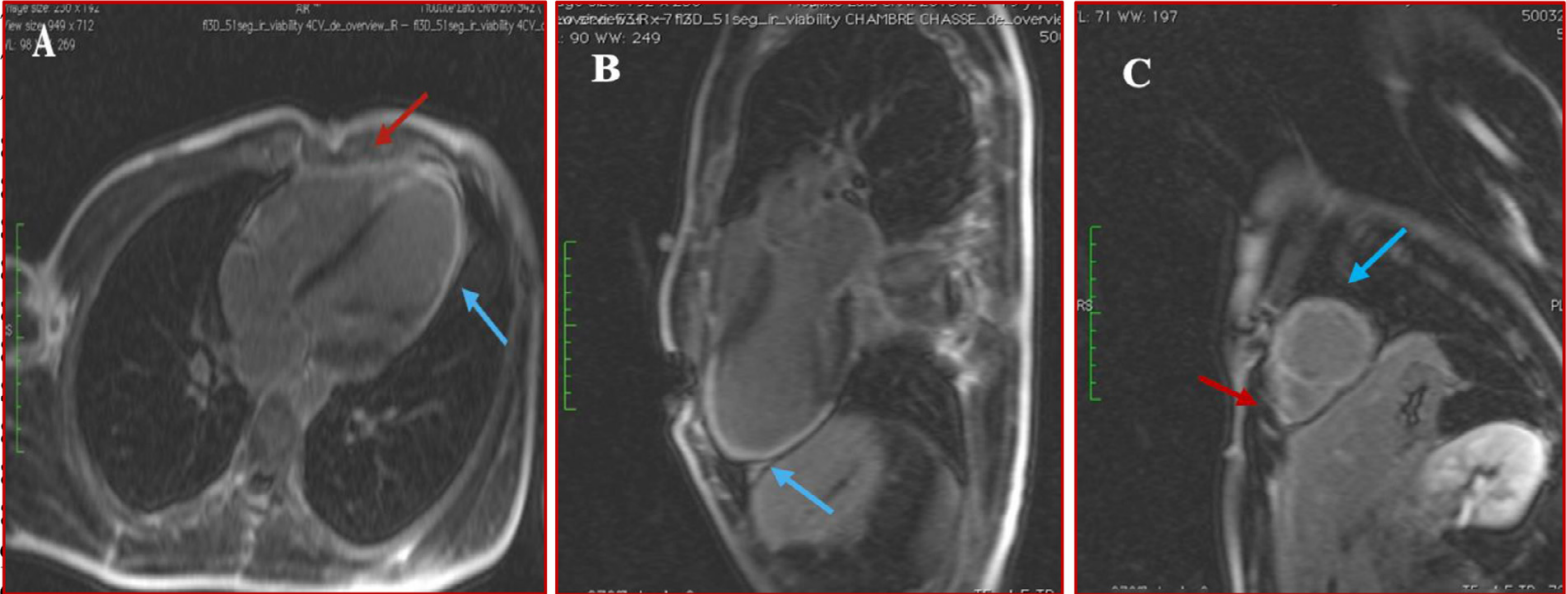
Phase sensitive inversion recuperation MRI sequences revealing progression of late gadolinium enhancement with transmural pattern (blue arrow) in lateral wall in the 4-chambers view (Image A); in the anterior and inferior wall (blue arrows) in the 2-chambers view (Image B) and in the apex in the short axis view (blue arrows) (Image C), with transmural enhancement of inferior and lateral walls of the right ventricle (red arrows).
